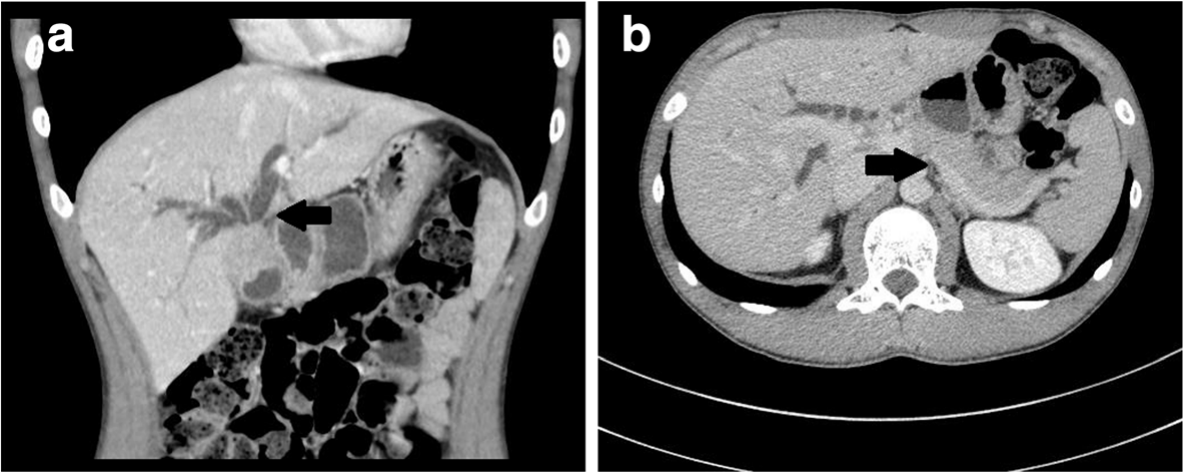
Pathologic findings on CT. a CT shows abnormal thickening of the gallbladder wall that appeared to invade the adjacent portion of the liver. An infiltrative low-density mass (30 mm) involving the gallbladder neck, upper biliary tract, and hilar bile duct as well as intrahepatic bile duct dilatation are visible (annotated with a *black solid arrow*). b The pancreas was not enlarged (annotated with a *black solid arrow*)
